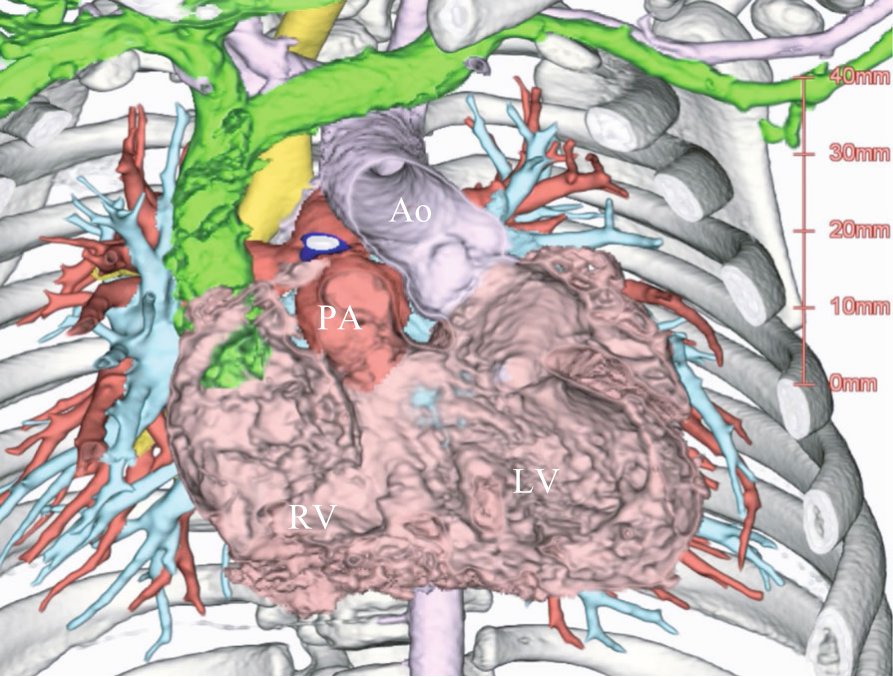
Preoperative computed tomography image showing the patient’s anatomical condition. Abbreviations: Ao, aorta; PA, pulmonary artery; RV, hypoplastic right ventricle; LV, left ventricle
